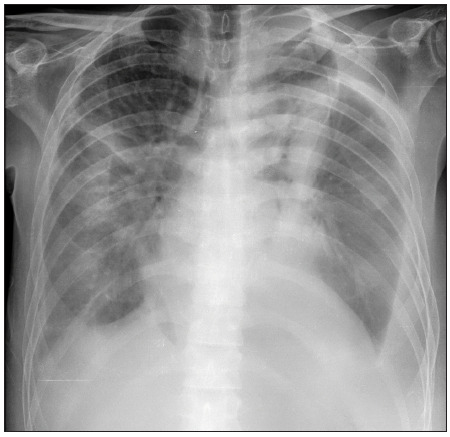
Lung radiograph from the second report, indicating pneumonia caused by *Bordetella bronchiseptica*.
